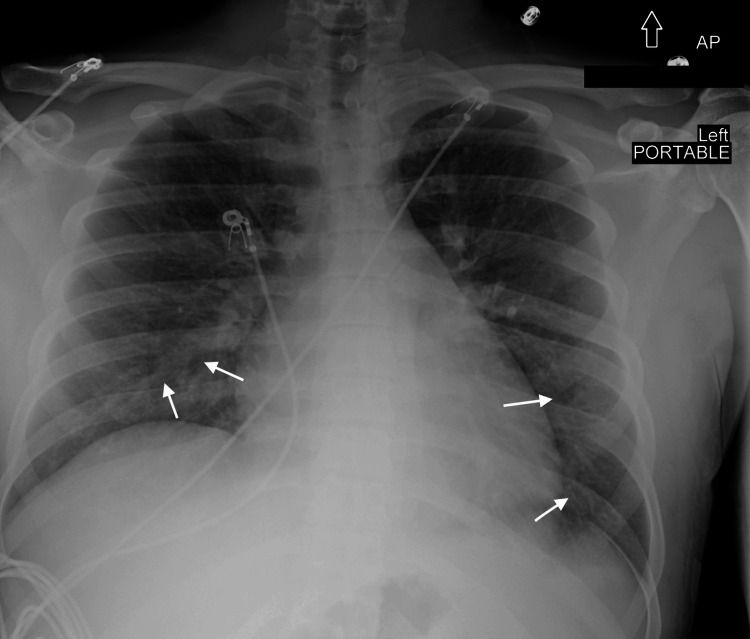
Anteroposterior chest X-ray showing bilateral patchy infiltrates (white arrows)
